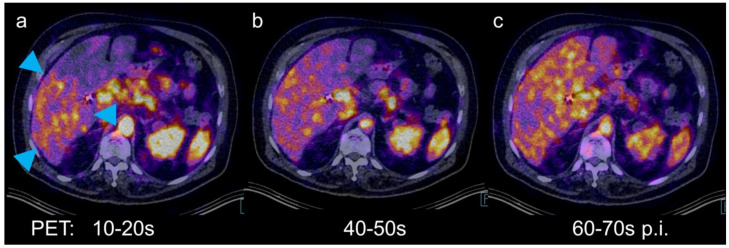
Positron emission tomography (PET)/CT has dynamic imaging capabilities for the depiction of liver tumors [3]. Therefore, an examination with the hepatobiliary tracer [68Ga]Ga-tri-methoxysalicyl-(TMoS)-DAZA was performed. Similar to mebrofenin derivatives and liver-specific MR contrast agents (e.g., gadoxetic acid), this 1,4-diazepan-6-amine (DAZA) ligand is extracted from the blood by hepatocytes and secreted into the bile [4,5,6]. In this case, 155 MBq of the tracer were injected intravenously. A dynamic PET of the liver over 3 min was acquired and reconstructed in 10-s timeframes. Early arterial hyperperfusion was evident in the region of the tumor (a, arrowheads). Tumor uptake was equal to normal liver 50 s after injection (b). From 60 s after injection, uptake in the tumor was lower than in the surrounding liver tissue (c).
